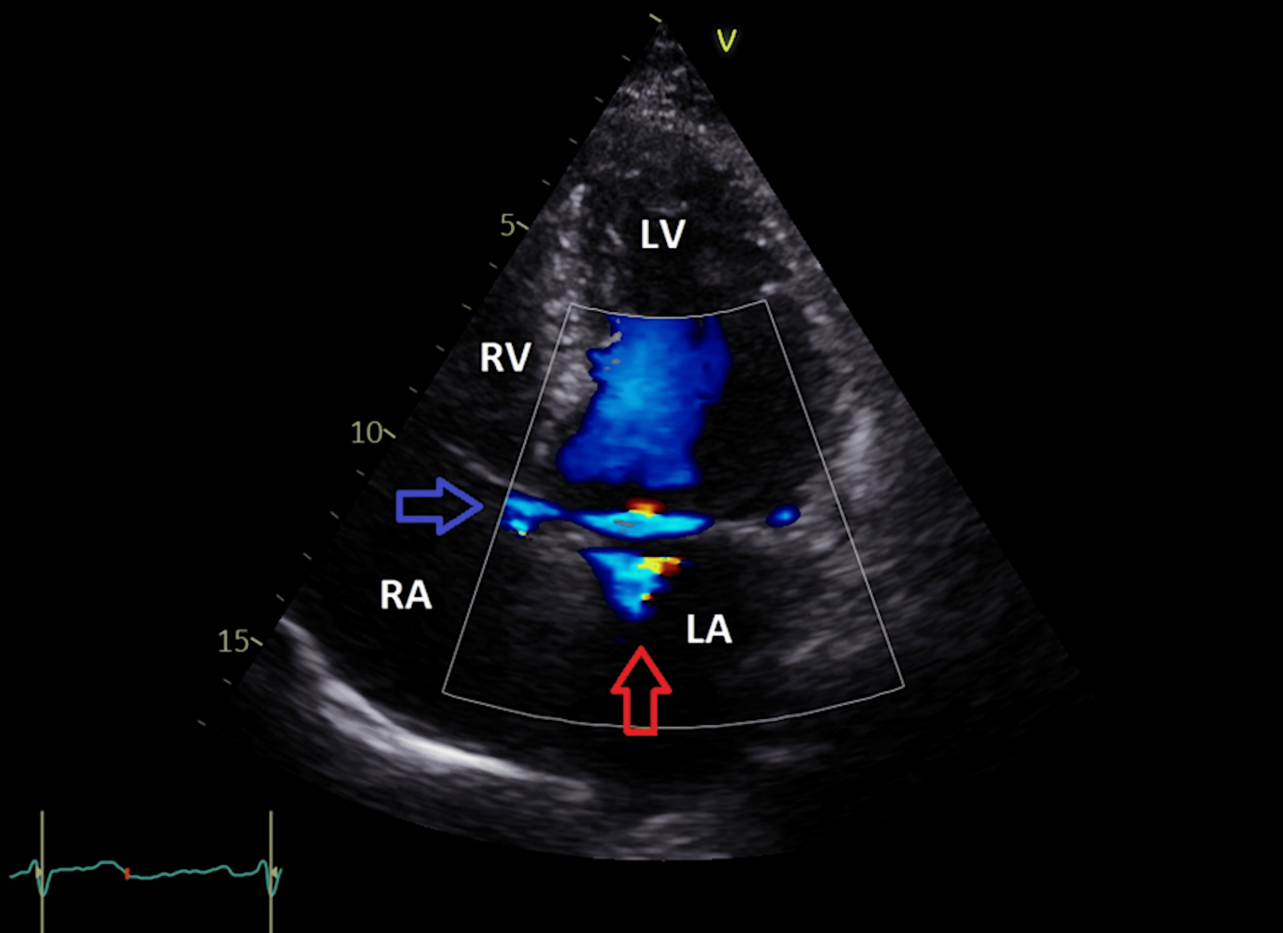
Apical four-chamber view showing mitral regurgitation jet (red arrow) with color aliasing in the right atrium (blue arrow). LA = left atrium; LV = left ventricle; RA = right atrium; RV = right ventricle
